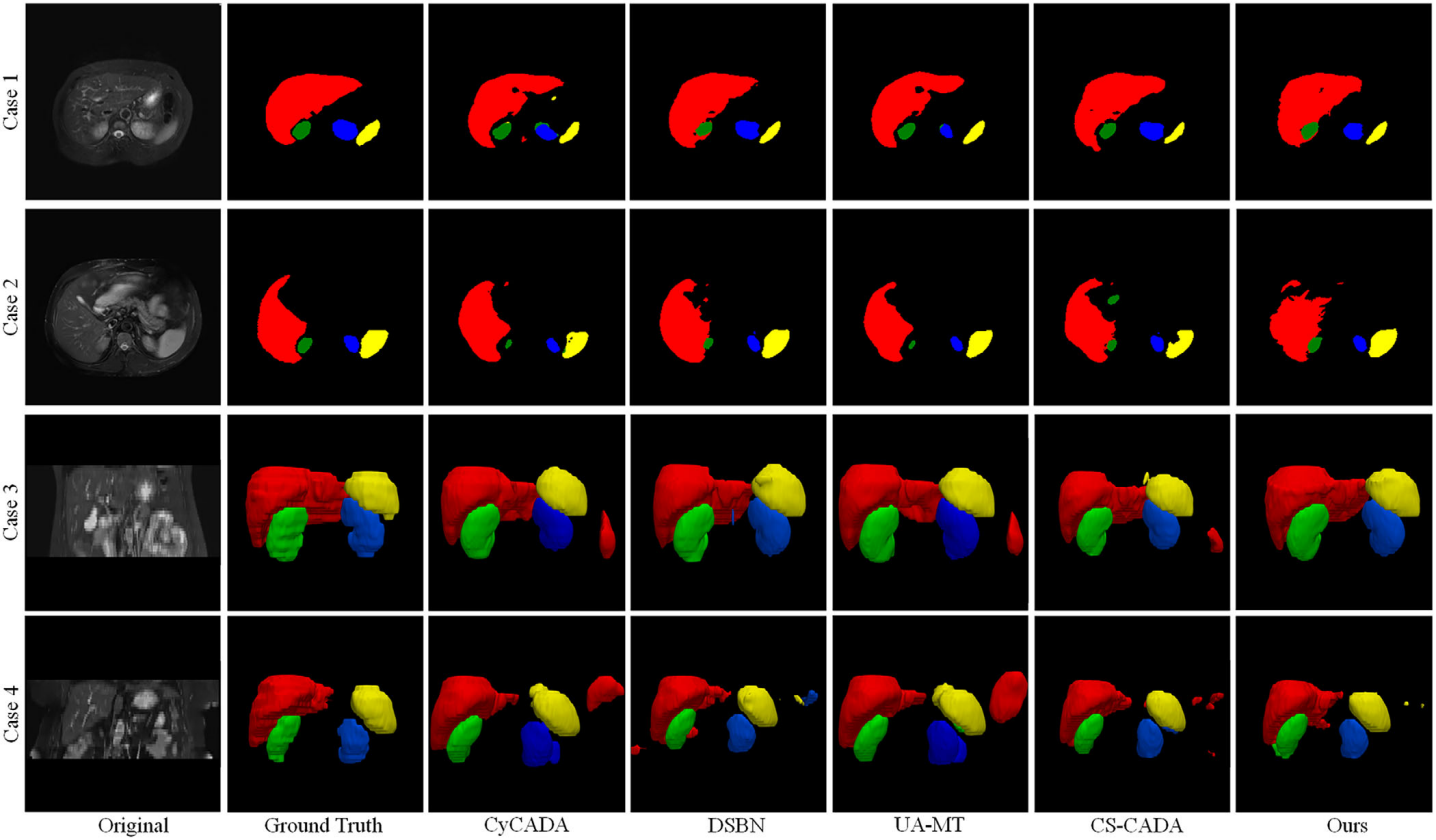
Comparative visualization of magnetic resonance imaging (MRI) segmentation in domain adaptation from computed tomography (CT) to MRI. The liver, the right kidney, the left kidney, and the spleen correspond to red, green, blue, and yellow, respectively.
